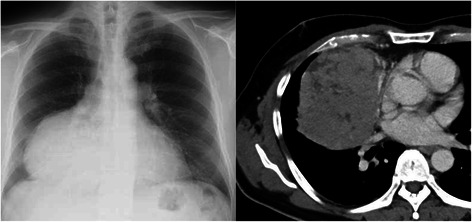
Chest x-ray shows a giant mass in the right lower lung field (*left*). Chest CT shows a mass measuring 13 x 10 cm in diameter, in contact with the right inferior pulmonary vein (*right*)
